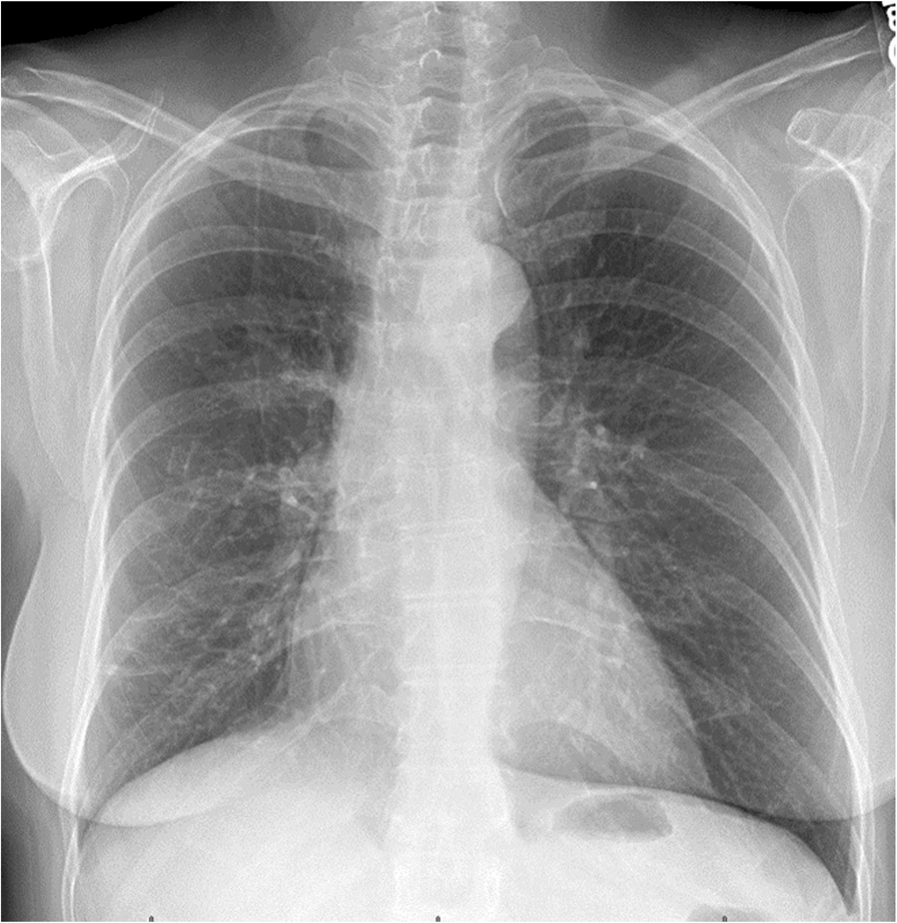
Chest X-ray showing resolution of pulmonary infiltrates and pleural effusion 1 month following steroid treatment
